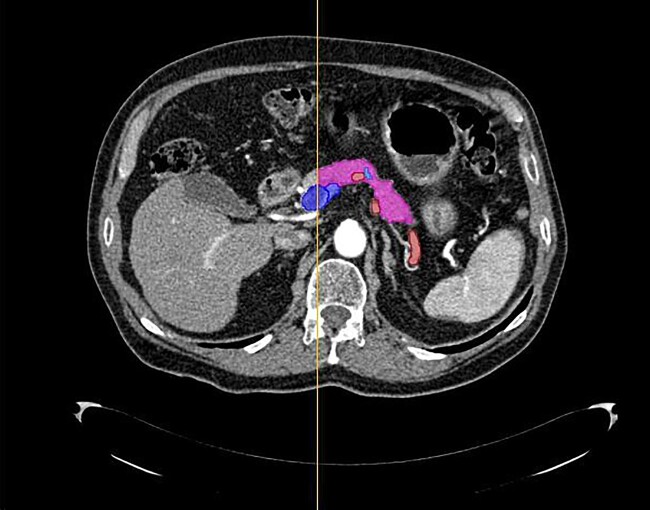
An example of a contrast-enhanced CT scan in the early arterial phase of the abdomen, showing the contoured pancreatic tissue (pink), pancreatic duct (light blue), splenic artery (red) and the superior mesenteric vein (dark blue) in an axial image The vertical yellow line indicates the midline of the superior mesenteric vein, with the pancreas annotated on the left side. The volume of interest consisted of the pancreatic tissue and pancreatic duct. CT, computed tomography.
